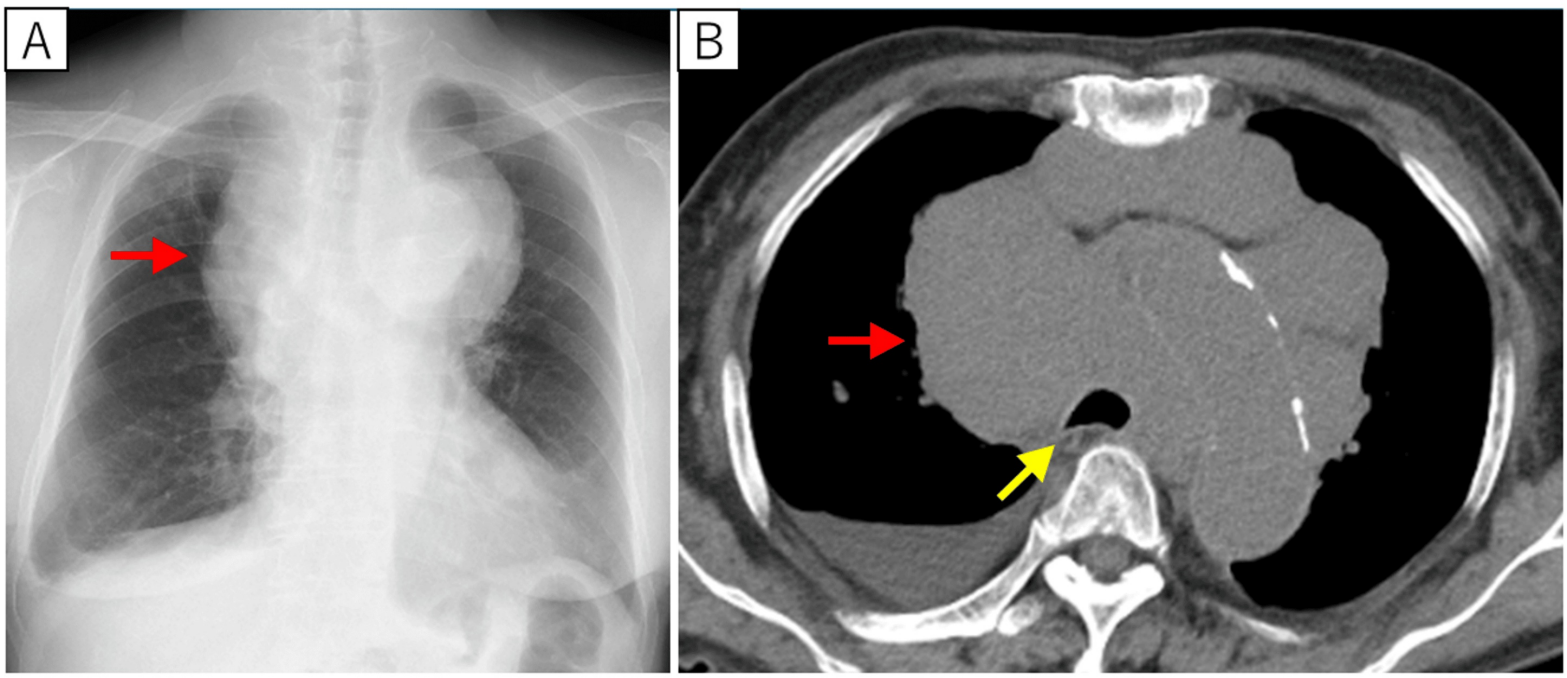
Chest imaging obtained at the onset of superior vena cava syndrome and respiratory symptoms On the chest X-ray (A), a new mass approximately 14.5 cm in length was found in the mediastinum (red arrow). On the chest CT, a mass was found in the mediastinum (red arrow), and the trachea was compressed and narrowed (yellow arrow) CT: computed tomography
